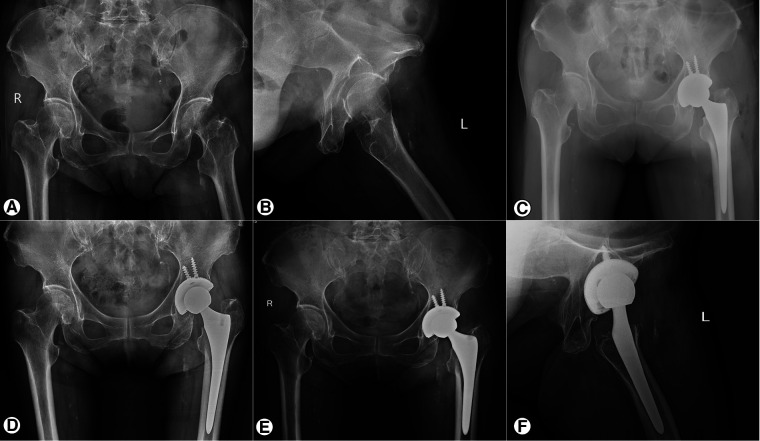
X-ray of a 73-year-old female FFN patient before and after THA. (A, B) The anteroposterior view of the pelvis and oblique view of the left hip before operation; (C) The anteroposterior view of the pelvis on the first day after operation. (D) Anteroposterior view of the pelvis 12 months after operation show that the prosthesis is well fixed. (E, F) The anteroposterior view of the pelvis and oblique view of the left hip at 42 months postoperatively. The prosthesis is well fixed and there is no lucent line between the acetabular prosthesis and the host bone.
